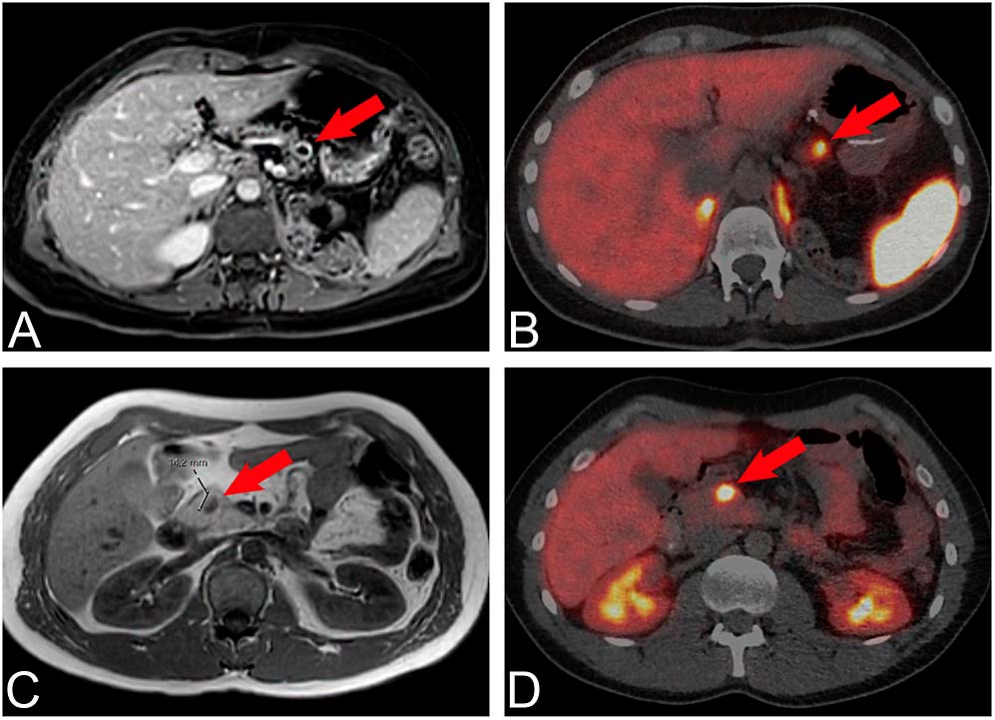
MRI scans and [^68^Ga]Ga-DOTATOC PET/CT scans of a cystic and solid pancreatic neuroendocrine tumour (PanNET). (A) MRI E-THRIVE image of a 14-mm cystic PanNET in the tail of the pancreas; (B) the corresponding [^68^Ga]Ga-DOTATOC PET/CT scan of the PanNET shown in A (SUVmax 13.1 and SUVmean 7.4). (C) MRI T1 image of a 14-mm solid PanNET in the head of the pancreas; (D) the corresponding [^68^Ga]Ga-DOTATOC PET/CT scan of the PanNET shown in C (SUVmax 13.7 and SUVmean 7.8).
